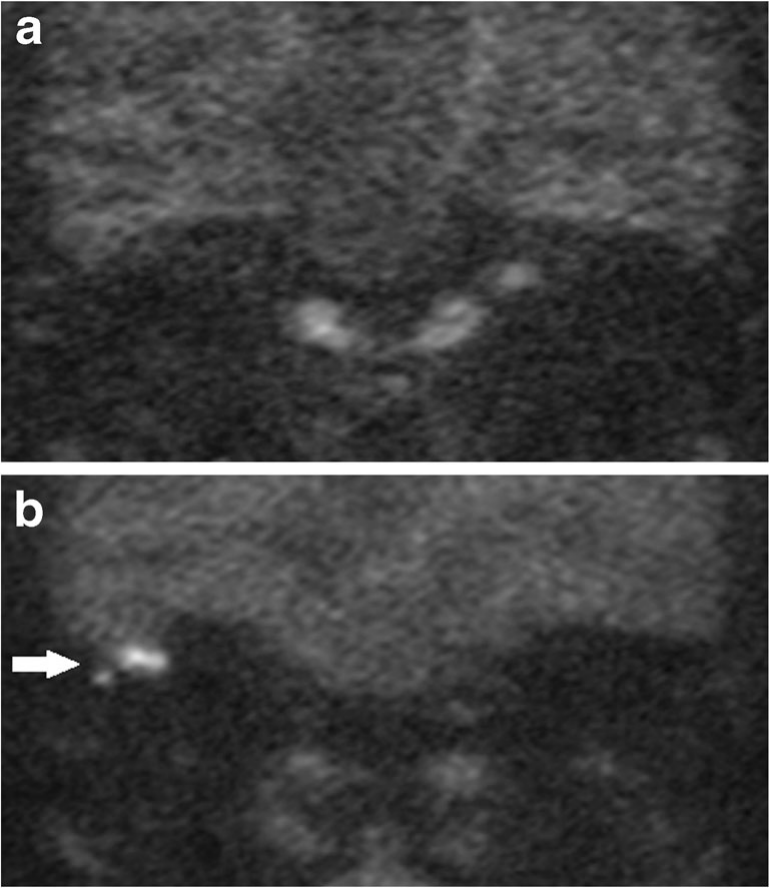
Value of monitoring DWI scans which are negative for the presence of post-operative cholesteatoma. A year following right canal wall up surgery, the DWI scan was negative as shown by the a coronal b1000 image. The patient opted for monitoring with DWI instead of second-look surgery as his ear was dry. b A follow-up DWI scan a year later showed residual cholesteatoma (*white arrow*), which was confirmed on subsequent surgery
